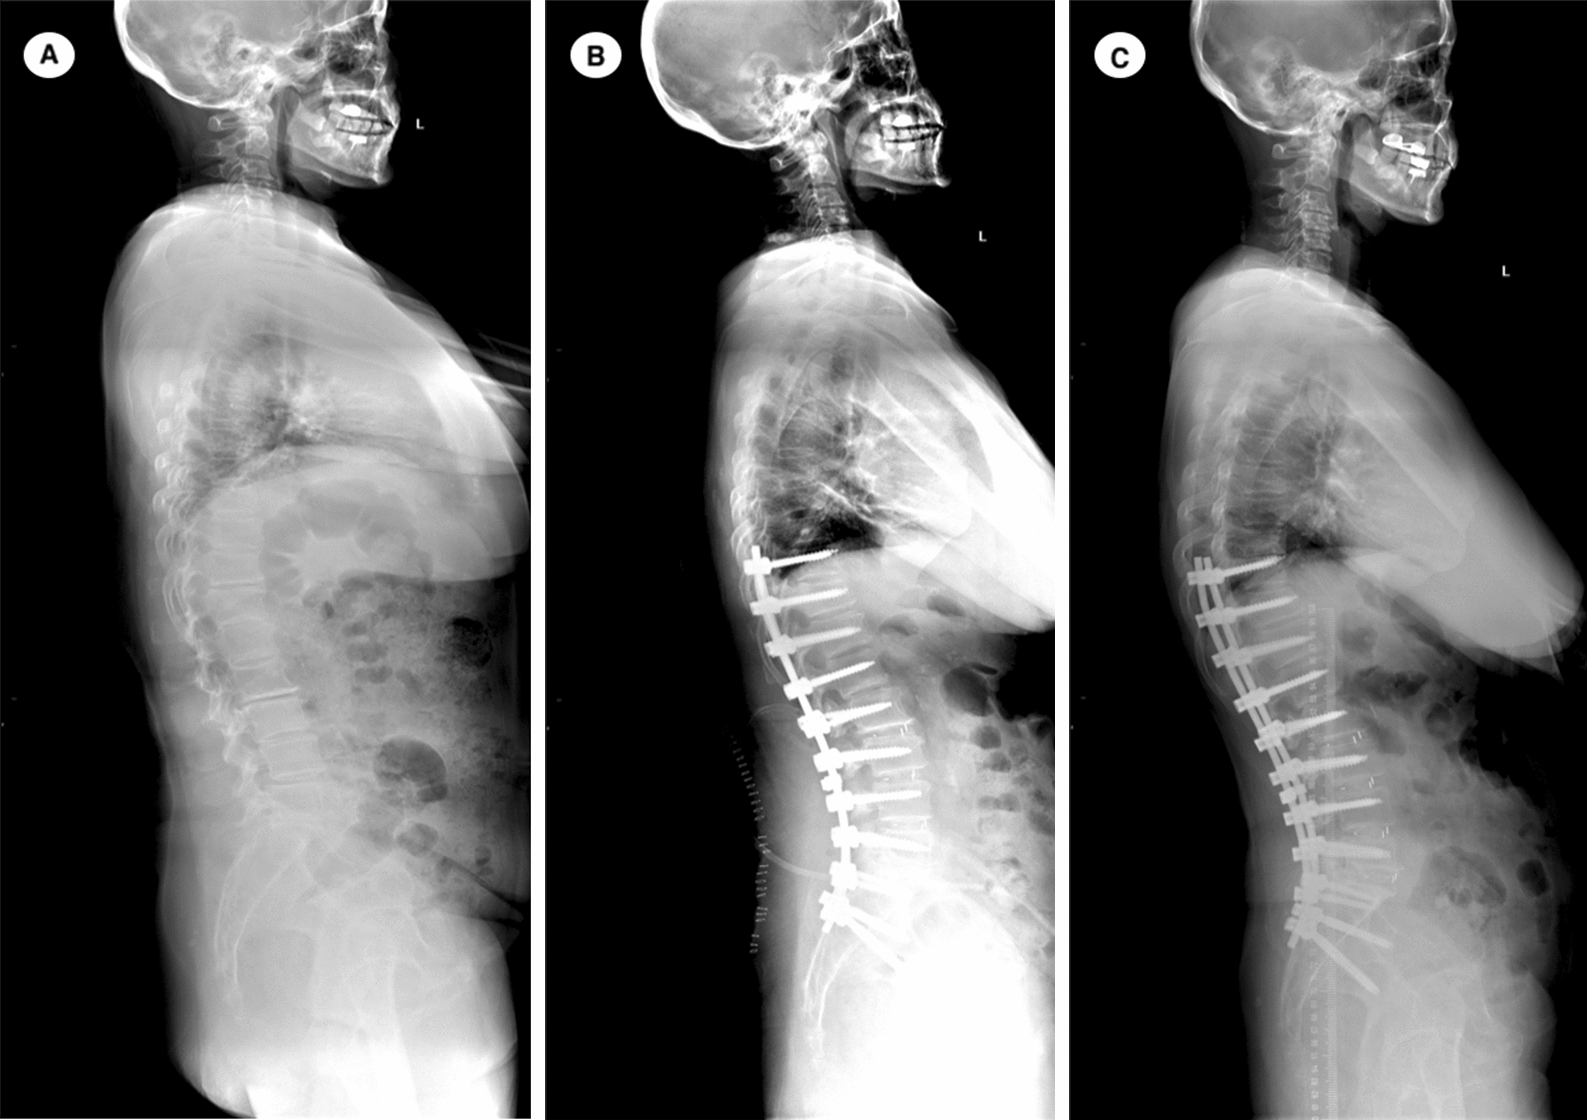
A 58-year-old female DLS patient underwent thoracolumbar fusion surgery (T10–S2). Radiographs show the changes in spinopelvic parameters, preoperative TK, LL, PT, SS, PI, PFA, SAA, SVA and TPA were 16.9°, −16.9°, 27.5°, 4.2°, 31.7°, 197.2°, 51.0°, −4.3 mm and 19°, respectively (A). Postoperatively, those variables were 37.6° for TK, -35.8° for LL, 20° for PT, 15.3° for SS, 35.3° for PI, 193.7° for PFA, 49.2° for SAA, −21.5 mm for SVA, and 14° for TPA (B). The PI–LL postoperatively was -0.5° at the post-operation. The patient has a significant upright posture after the surgery (B); however, PJF developed at the 18^th^ month during follow-up (C)
